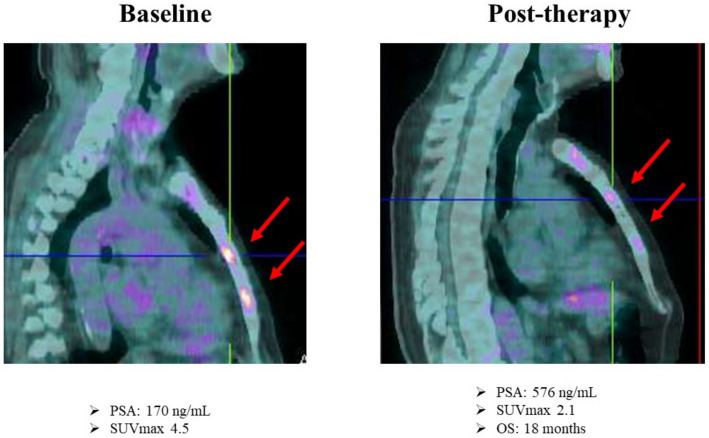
18F-FDG PET at baseline and after three cycles of Ra-223. The red arrows indicate metabolically active bone lesions.
